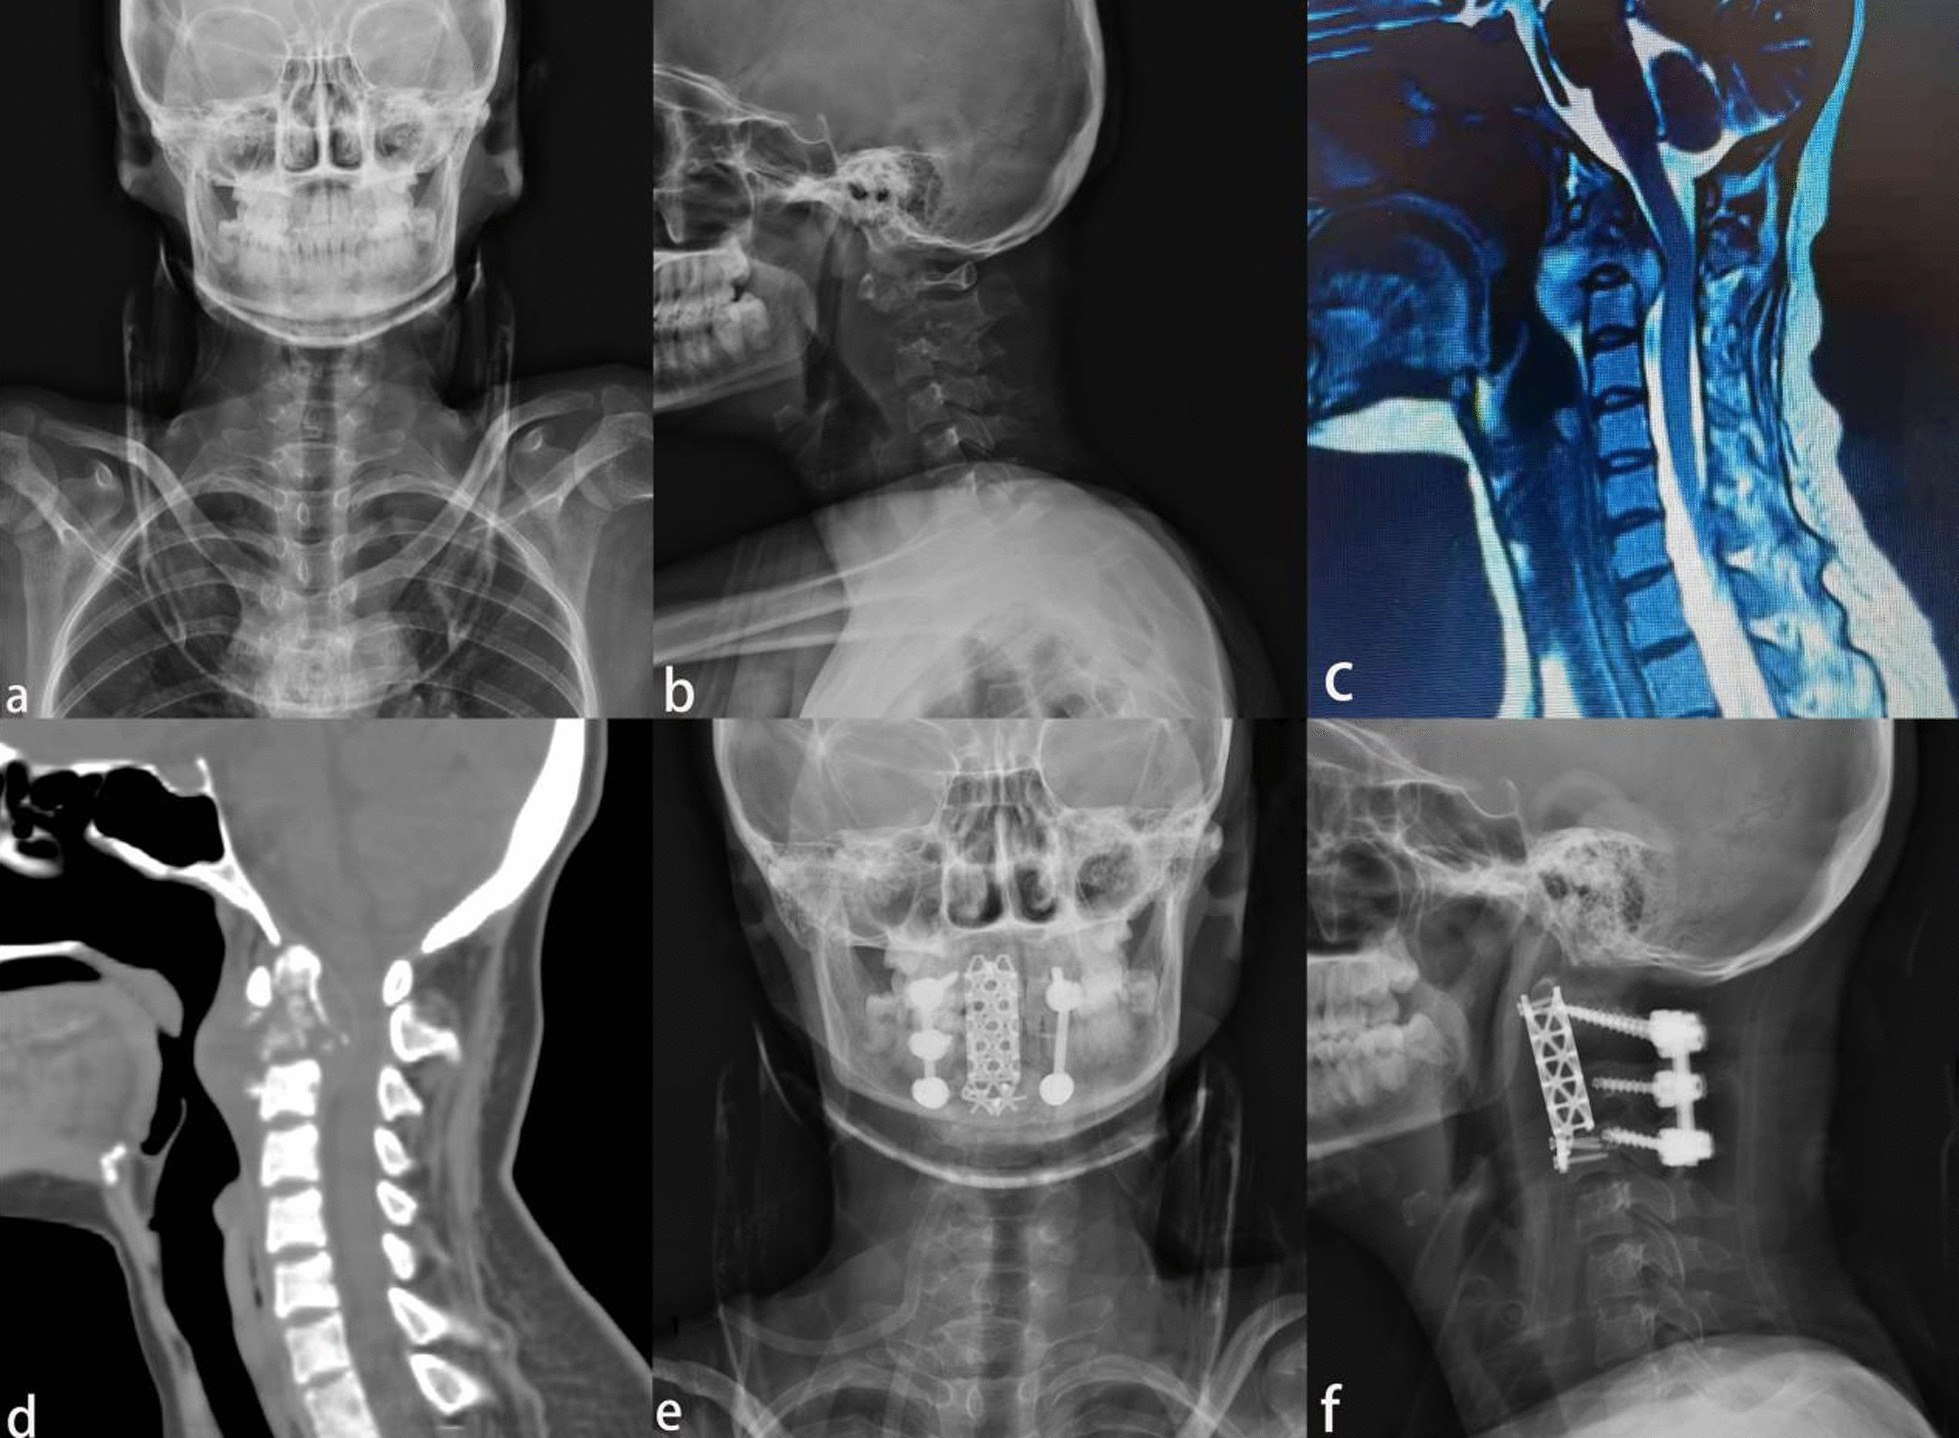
A 23-year-old woman with a 3-month of limited neck pain and mobility and 1 week of progressive weakness of the extremities. a, b Anteroposterior and lateral X-ray showed C1 and C2 vertebral destruction and local kyphosis from C1 to C3. c Cervical MRI showed significant loss of C2 vertebral body height, local kyphosis, compression of the sac, and localized prevertebral abscess. d Cervical CT showed C1 and C2 vertebral destruction, intraspinal encroachment, and local kyphosis from C1 to C3. e, f Postoperative anteroposterior and lateral X-ray showed C2 and partial C3 vertebrectomy, vertebral body reconstruction using titanium cage, and posterior approach surgery using cervical 2 pedicle screw and cervical 3, 4 lateral mass screw fixation
